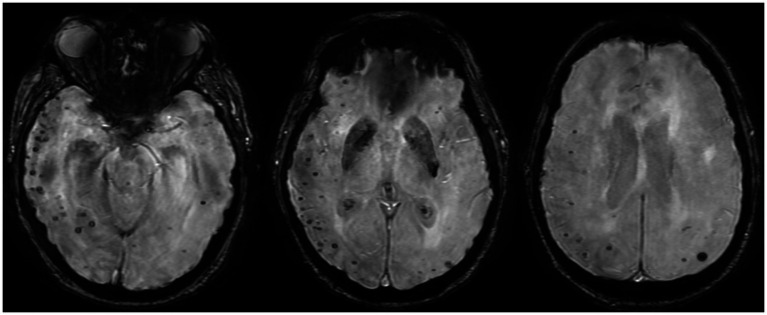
Susceptibility-weighted magnetic resonance imaging with three axial cross-sections demonstrates numerous bihemispheric punctate foci of susceptibility, especially in the right temporo-parieto-occipital lobes, suggesting microbleed.
